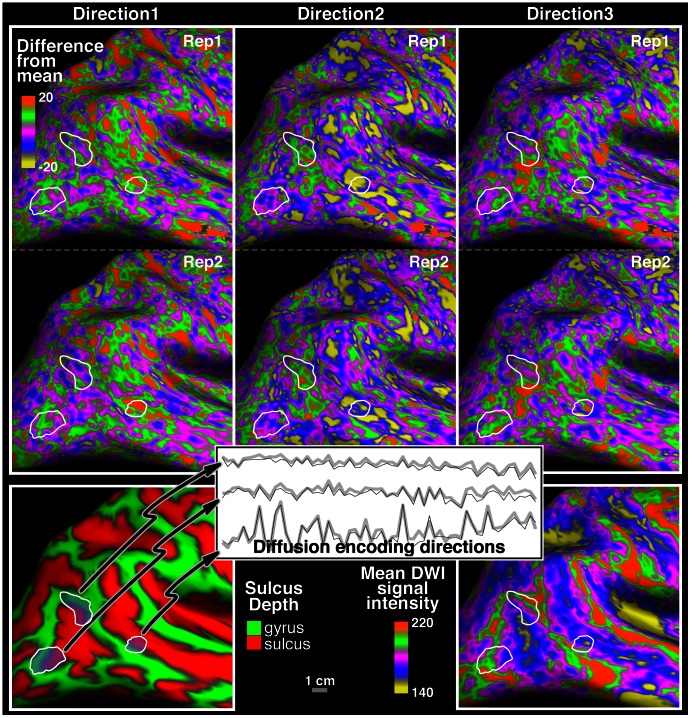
Test/re–test DWI signal intensity. The top two rows display the raw diffusion weighted image (DWI) signal intensity after the mean signal of all the 61 DWIs (see bottom right) has been subtracted from each. Three diffusion–encoding directions of both repetitions (top = Rep1, middle = Rep2) are shown. The white outlines indicate the ROIs from Figure 1. The bottom right displays the mean of the 61 DWIs. The color bar represents MRI image pixel intensity from yellow = low to orange = high. Note the different ranges between the individual images and the mean. On the bottom left gyrification of the cortex is indicated (red = sulci, green = gyri). The inset signal time–courses in the centre of the figure depict how the pixel intensity varies over the entire experiment of 61 diffusion–encoding directions within the 3 ROIs (Rep 1 = thick grey line, Rep 2 = thin black line). The scale for all images is 1 cm.
